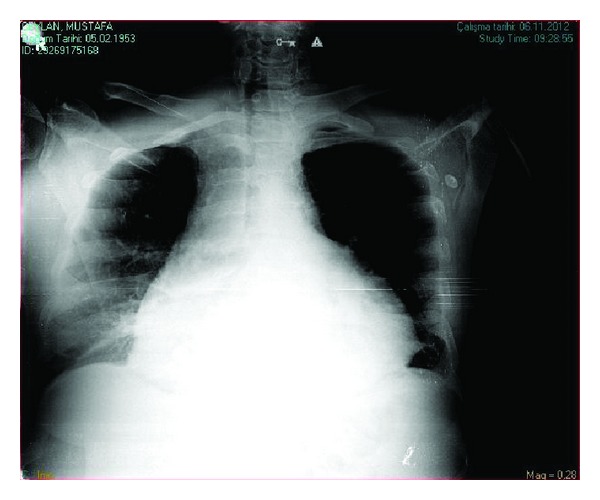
Chest X-ray demonstrating cardiomegaly with pericardial effusion.
